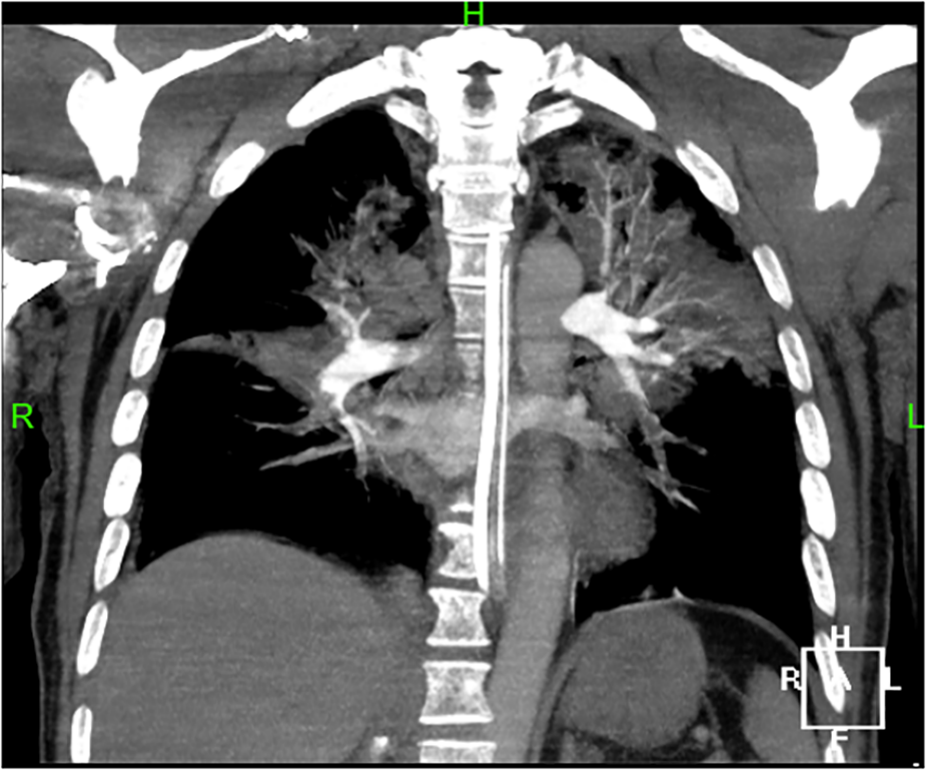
Pulmonary artery CTA shows bilateral lower pulmonary embolism, involving multiple segmental branches.
